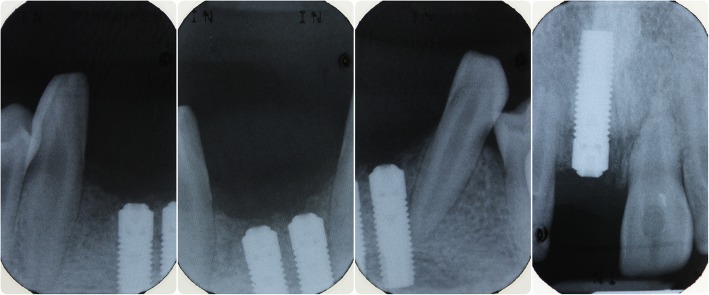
Placement confirmation. Radiograph of fixtures in 11, 31 and 41 positions confirming angulation
